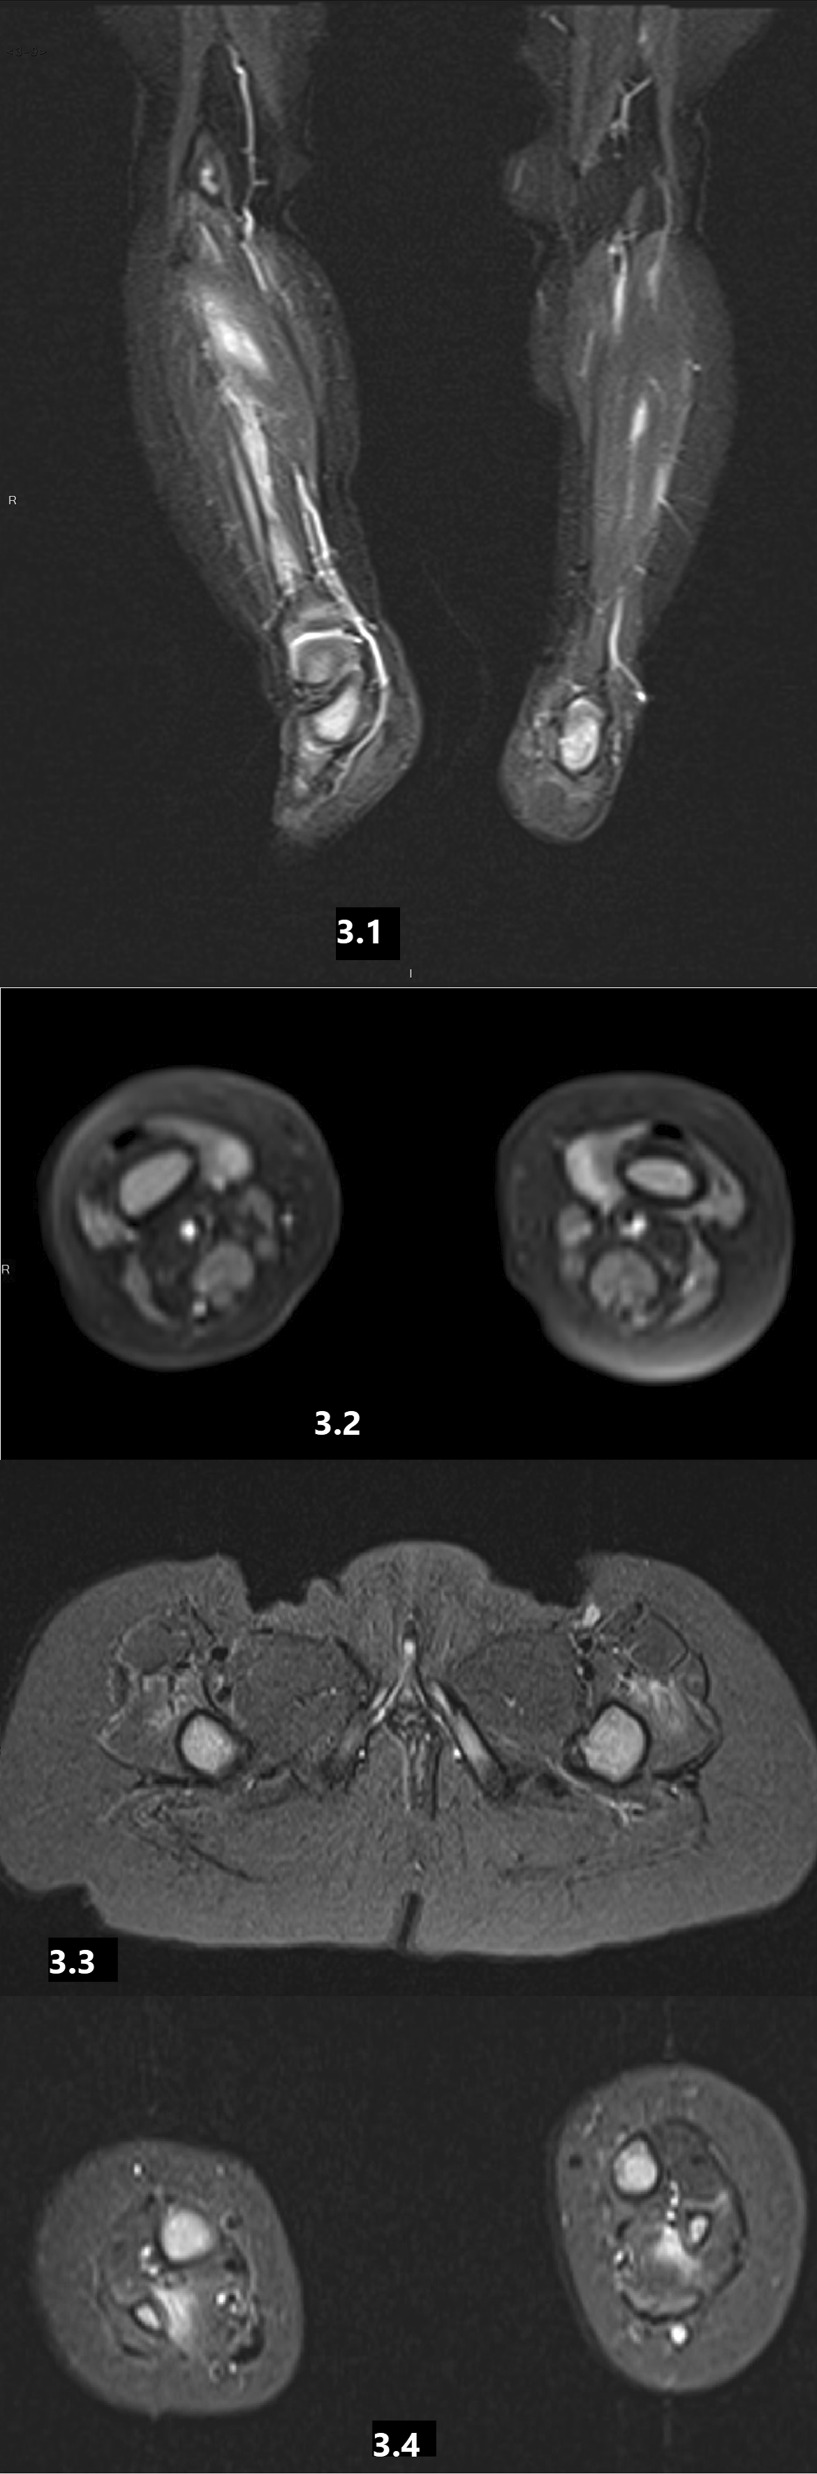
MRI of the lower limbs (Images 3.1, 3.2, 3.3, 3.4) of patient number 3, reveals extensive edema involving the muscles without fat atrophy, compatible with myositis
